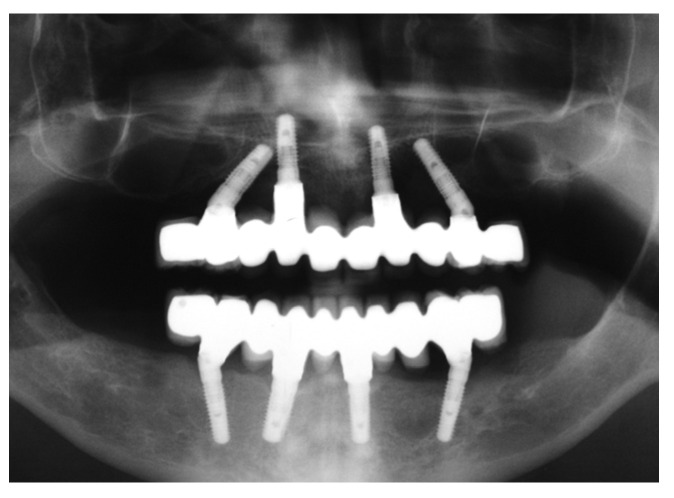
Orthopantomograph obtained after the delivery of the definitive prosthesis.
